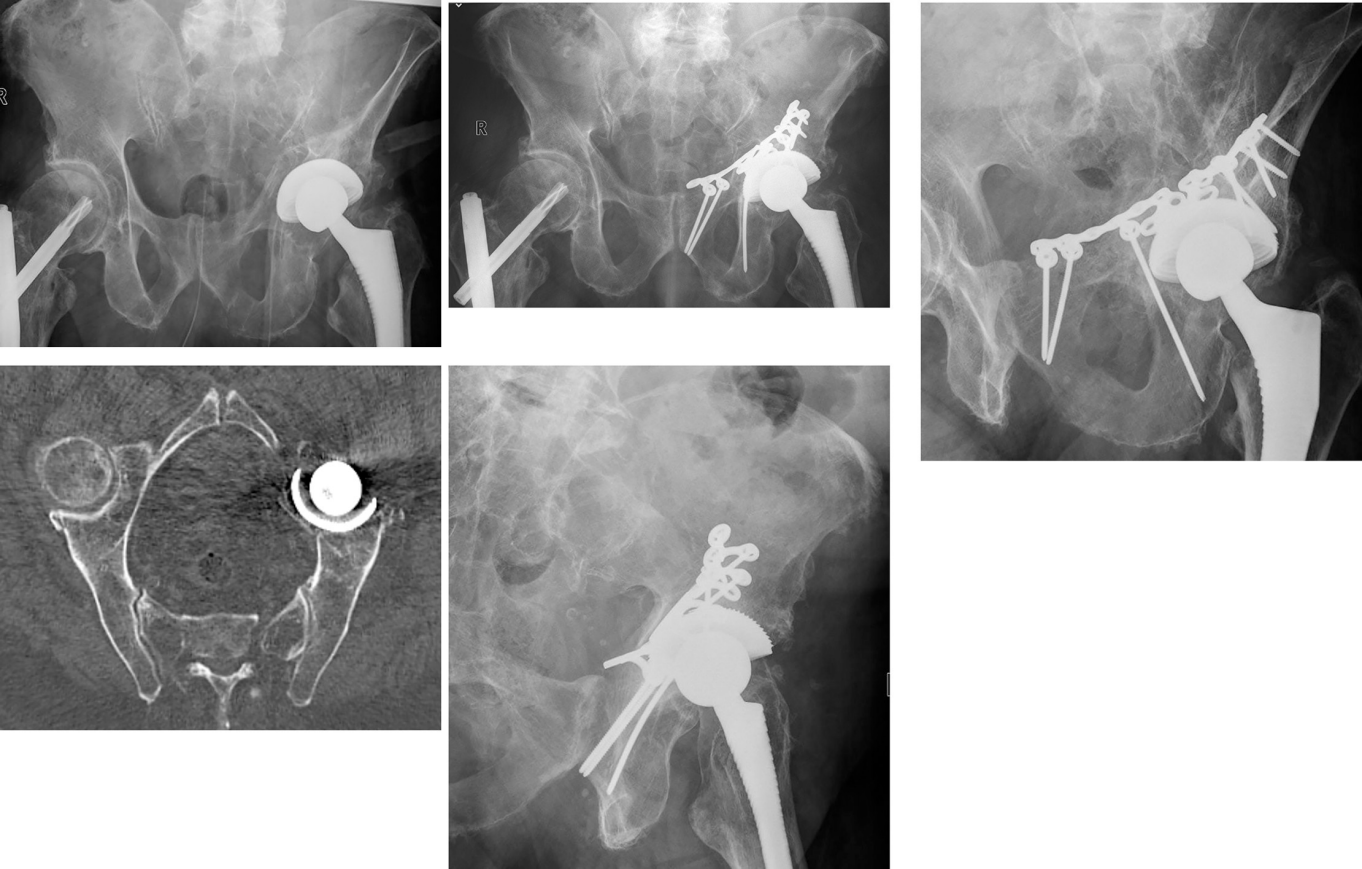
Seventy-eight old man with periprosthetic acetabular fracture after fall. The left hemiprosthesis has been inserted 9 years earlier. (a) Pelvic ap overview. (b) CT reconsruction at the level of the pelvic brim. The medial displacement of the quadrilateral plarte is clearly visible. (c) Pelvic overview 2 months after ORIF. The quadrilateral surface has been reduced and fixed with an Omega plate. (d) Ala view. (e) Obturator view. The patient shows good mobility with a rolling walking frame.
